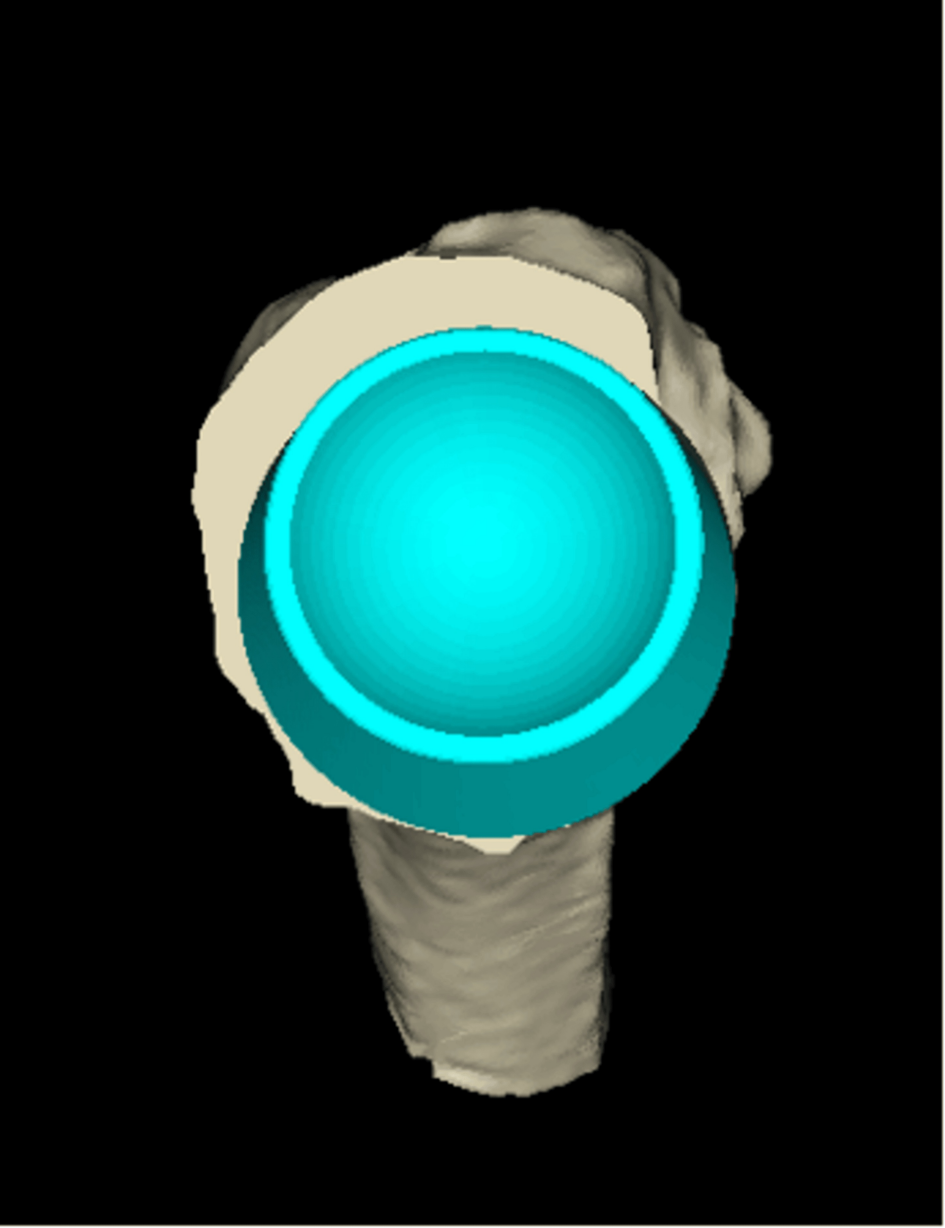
Sagittal 3D CT-based planning view demonstrating the humerus with a high-offset tray and +6 mm liner. The posterior positioning of the offset is shown, designed to counteract anterior soft-tissue tension and enhance joint stability in the setting of chronic anterior dislocation.
